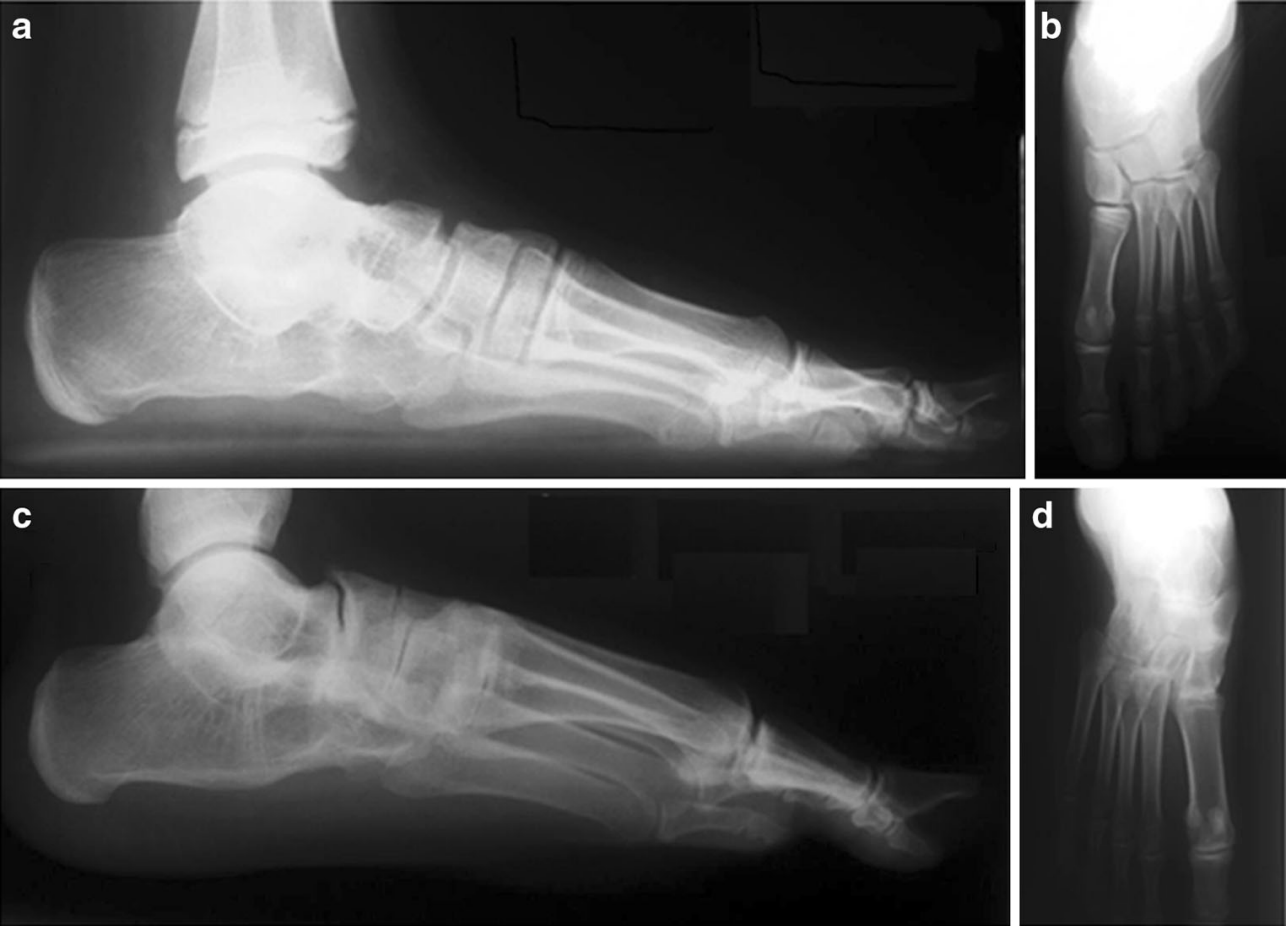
Radiographic examples of a complete union (a, b) and non-union (c, d) after a triple arthrodesis. The complete union radiographs were taken 1 year and 7 months after surgery in a male who had a triple arthrodesis surgery for a tarsal coalition at 13 years of age (a, b). The non-union radiographs were taken 2 years and 6 months after surgery in a male who had triple arthrodesis for a tarsal coalition and syndromic condition at 13 years of age (c, d)
